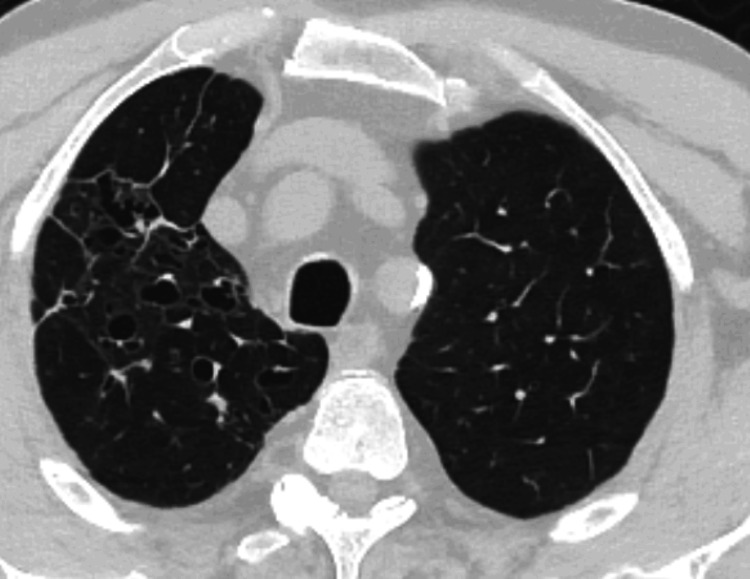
Follow-Up Chest Computed Tomography After ABPA Treatment ABPA, Allergic bronchopulmonary aspergillosis
